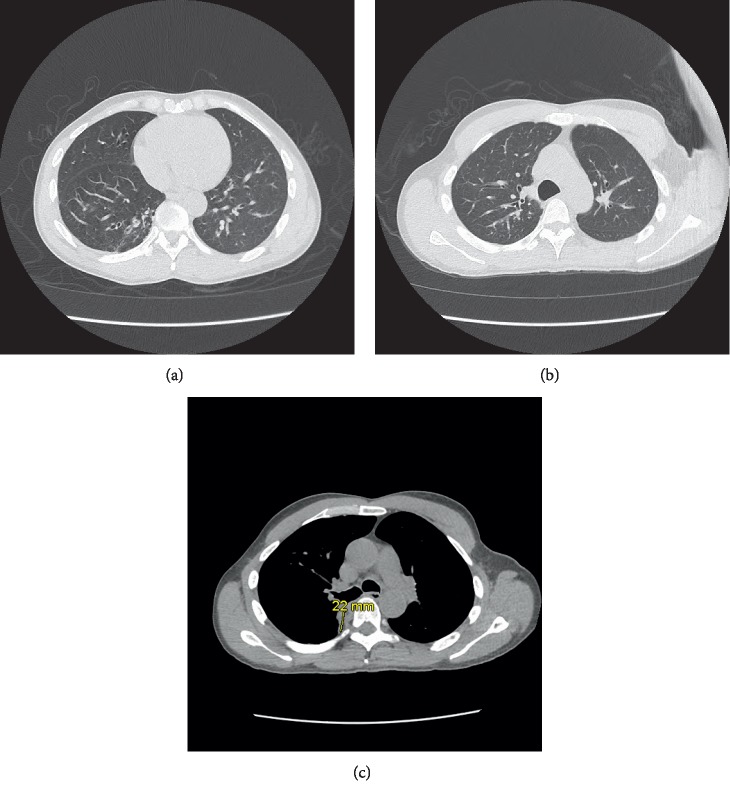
Chest CT without contrast revealing (a) bronchial wall thickening and bronchiectasis in the bilateral lower lobes; (b) bronchial wall thickening, bronchiectasis, and tree-in-bud nodularity; and (c) a 1.0 × 2.2 × 1.8 cm solid nodule in the posterior medial right lung.
